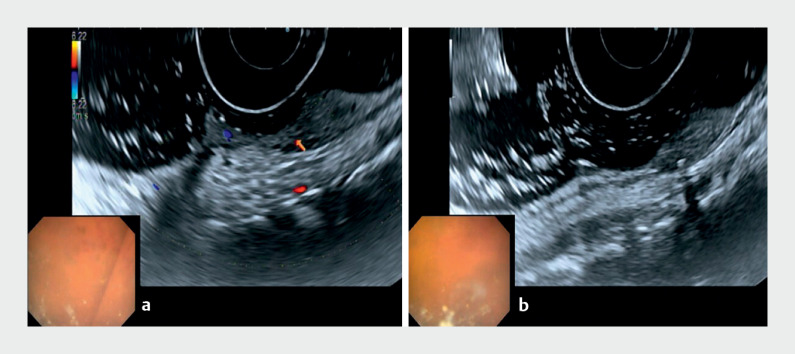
Endoscopic ultrasonographic assessment of the lesion.
